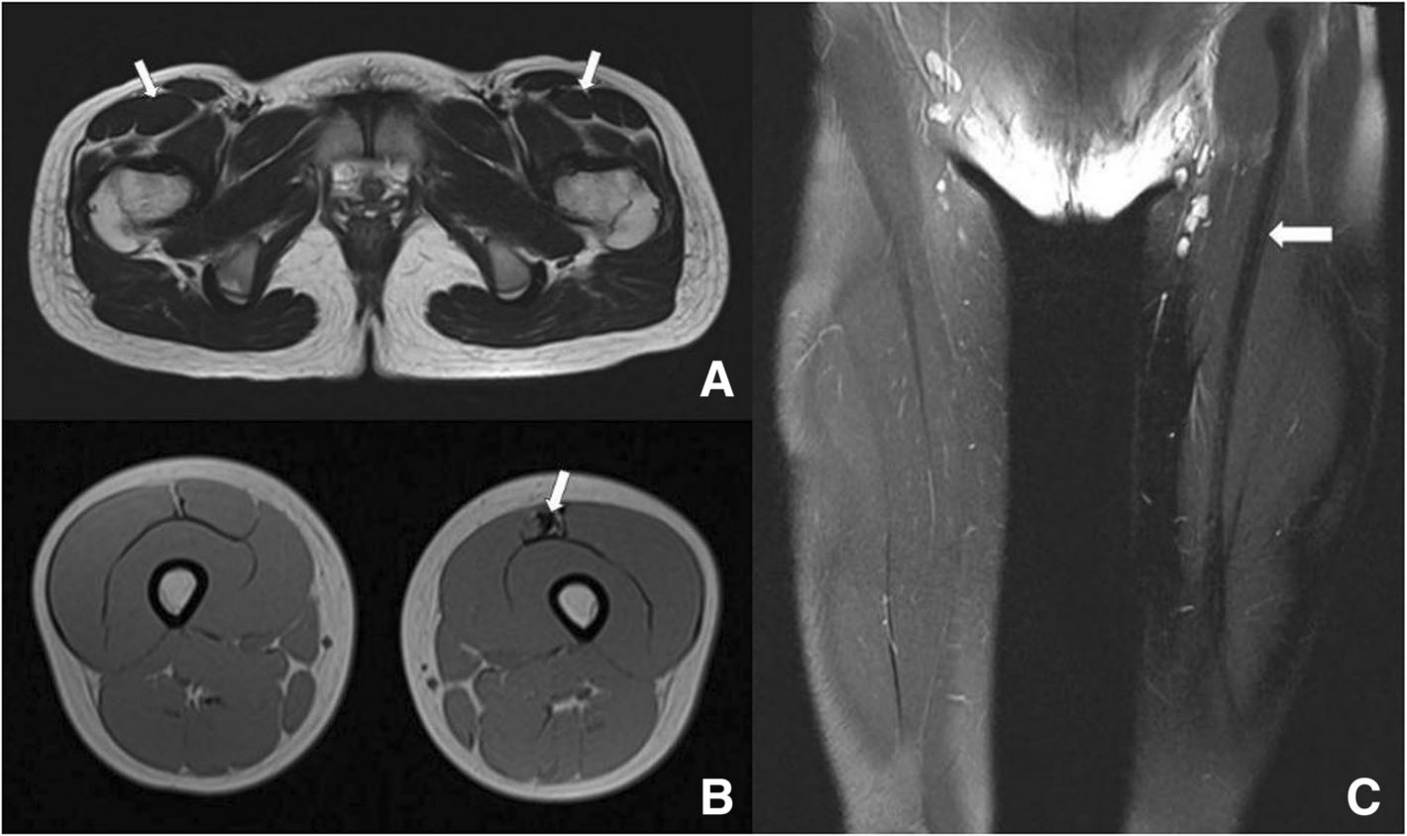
Preoperative MRI findings. Transverse T2-weighted image (a) showing volume reduction of left rectus femoris muscle. Transverse T1-weighted image (b) demonstrating fibrosis with dark signal intensity in the anterior of left rectus femoris. A hypointensity band could be observed on the coronal section (c) in the image. *White arrow in A*: rectus femoris muscle; *White arrow in B &C*: hypointensity band on the left rectus femoris muscle
